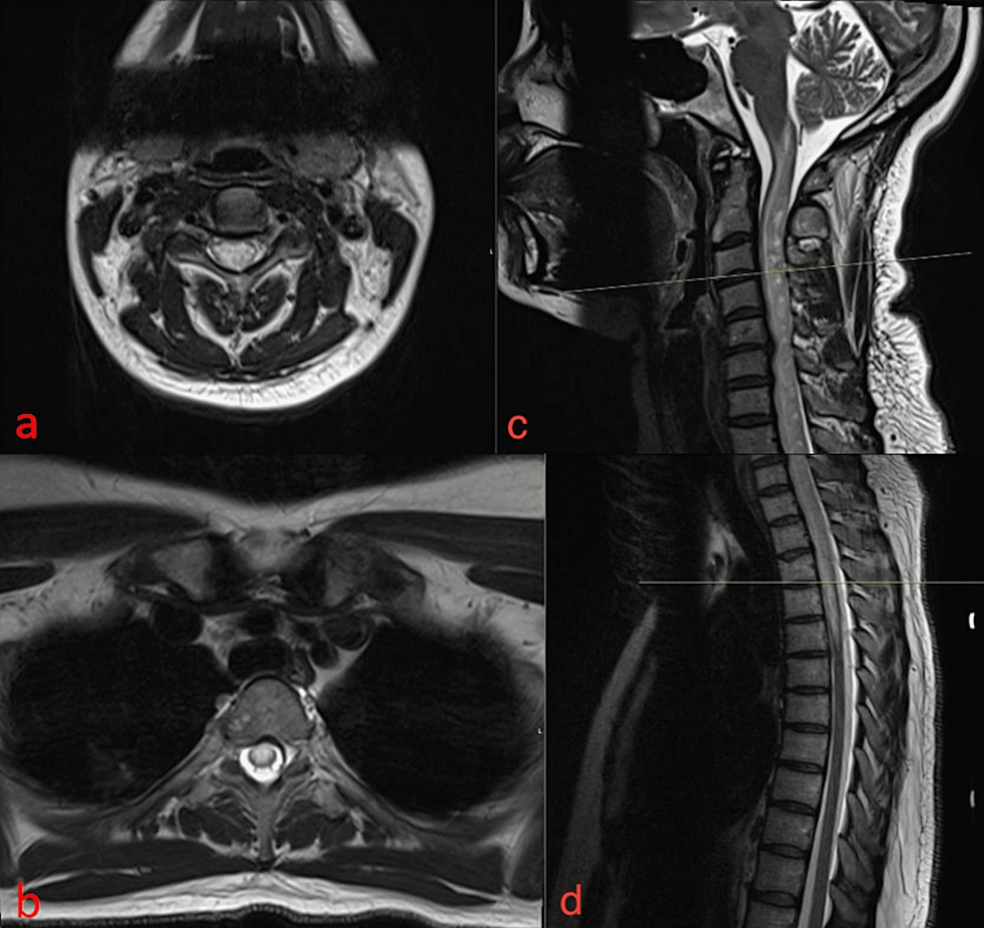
Axial localizer T2 weighted magnetic resonance images of the cervical (a) and thoracic (b) spine. Sagittal T2 (c and d) weighted magnetic resonance images of the cervical (c) and thoracic (d) spine. Axial images (a and b) show similar findings to sagittal (c and d) images, which are also included in Figure 1, with signal abnormality with enhancement of the posterior aspect of the spinal cord/adjacent dura.
